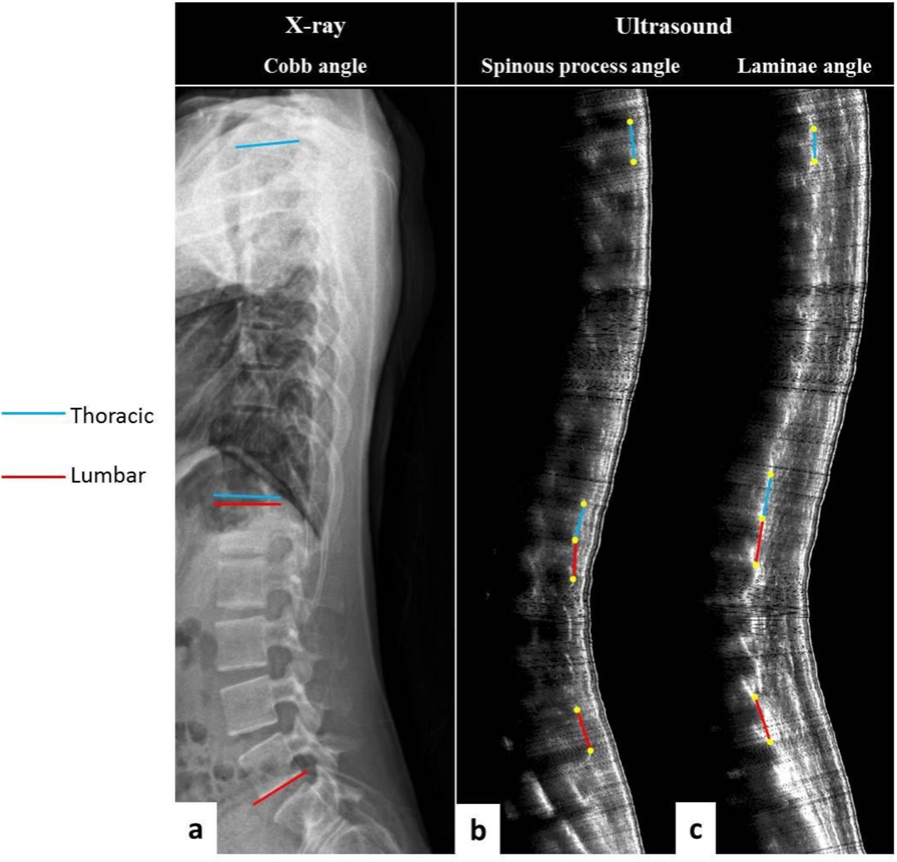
a) Measurement of X-ray Cobb’s angle (XCA); b) Measurement of ultrasound spinous process angle (USSPA); c) Measurement of ultrasound laminae angle (USLA) (Thoracic and lumbar angles are defined by the angle between the blue and red lines respectively)
